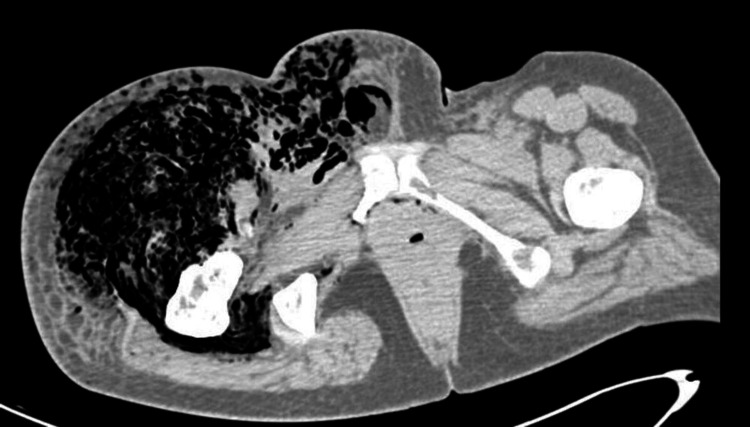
Axial CT scan of the pelvis showing extensive soft tissue edema and widespread gas collections dissecting through fascial and muscular planes in the right hemipelvis, indicative of tissue necrosis and gas-forming infection. The left hemipelvis shows preserved soft tissues.
